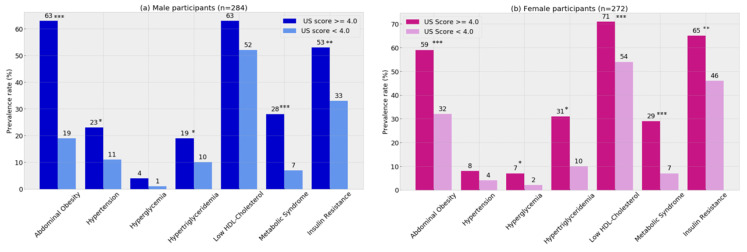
Cardiometabolic risk at 23y in the Santiago Longitudinal Study by NAFLD diagnosis with abdominal ultrasound: male (a) and female (b) participants Metabolic Syndrome and its components were diagnosed with the AHA/NHLBI/IDF joint standard. Insulin resistance was diagnosed with HOMA-IR values ≥ 2.6 in both male and female participants. Chi2 test for independence: * *p* < 0.05,  *p* < 0.01, * *p* < 0.001.
